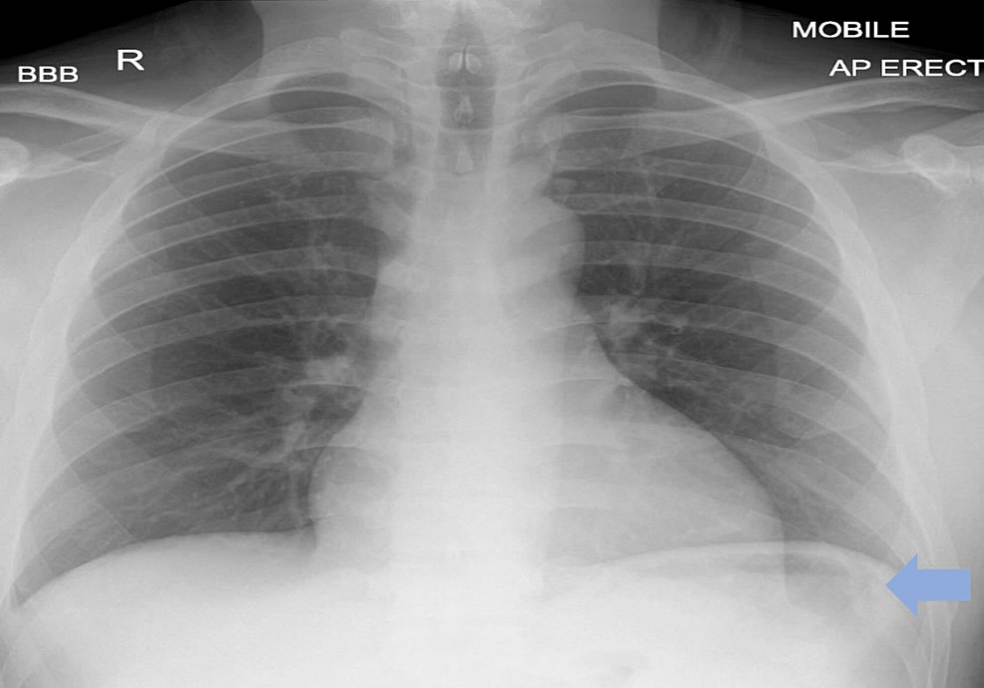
Repeat chest radiography shows a very small residual pneumoperitoneum.
